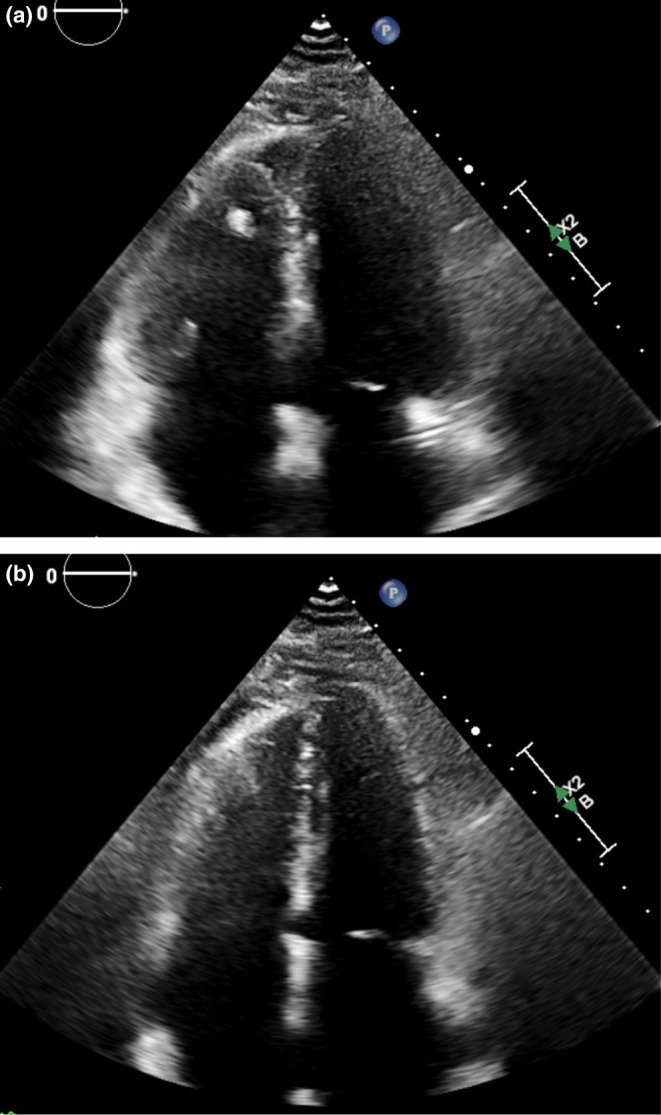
(a) Apical four‐chamber view during end‐diastole showing normal right and left ventricular chamber sizes and geometry without chamber dilation or septal flattening, consistent with the absence of right ventricular volume or pressure overload. (b) Apical four‐chamber view during end‐systole showing preserved systolic contraction without regional wall motion abnormality.
